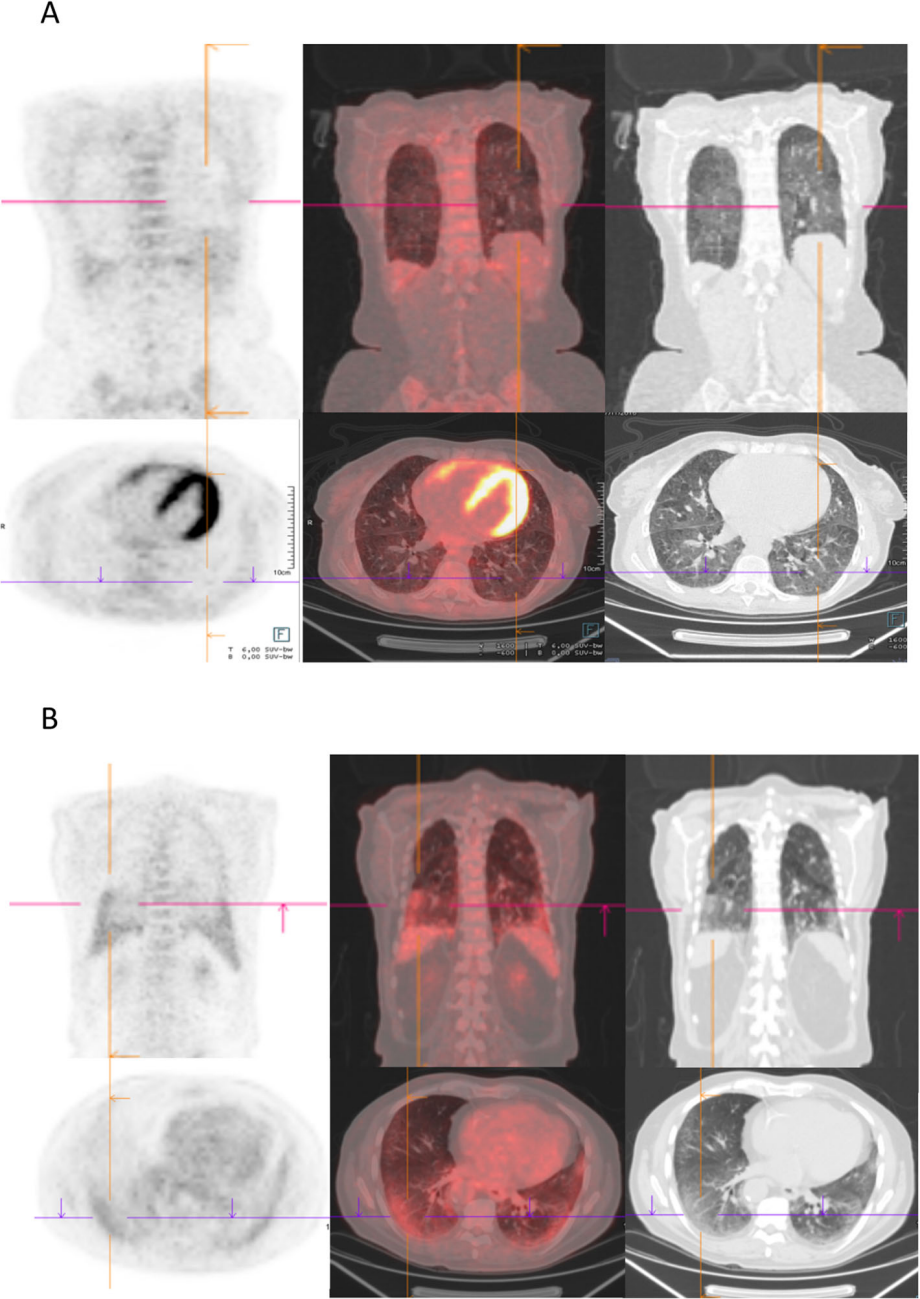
Visual examination of FDG PET/CT scans. Transverse slides of the FDG PET/CT scans of a a SSc-ILD with an abnormal interstitial lung pattern of FDG uptake and b a SSc-ILD without an abnormal interstitial lung pattern of FDG uptake
